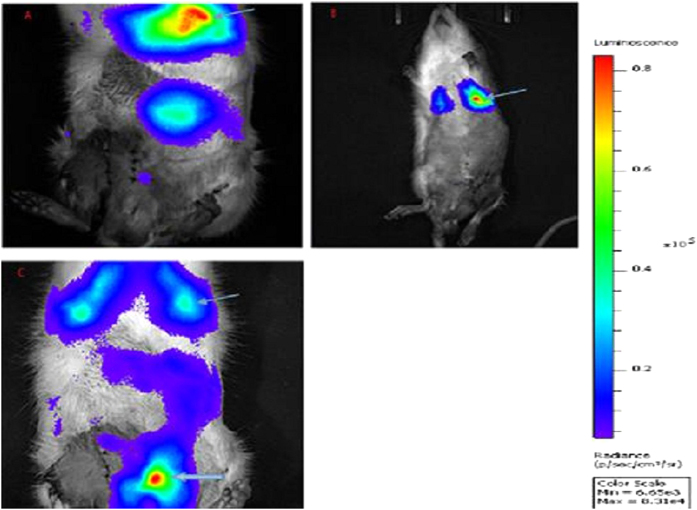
Distribution and amount of fluorescein-labeled BMMSCs in the different groups assessed by *in vivo* imaging. (a) In the ultrasound group, the fluorescein-labeled BMSCs were mainly found in the lung tissue (thin arrow) and epigastrium. (b) In the BMMSCs group, the fluorescein-labeled BMMSCs were found in the lung tissue (thin arrow). (c) In the MMUS group, more of the fluorescein-labeled BMMSCs were found in the prostate tissue than in the other groups (thick arrow).
